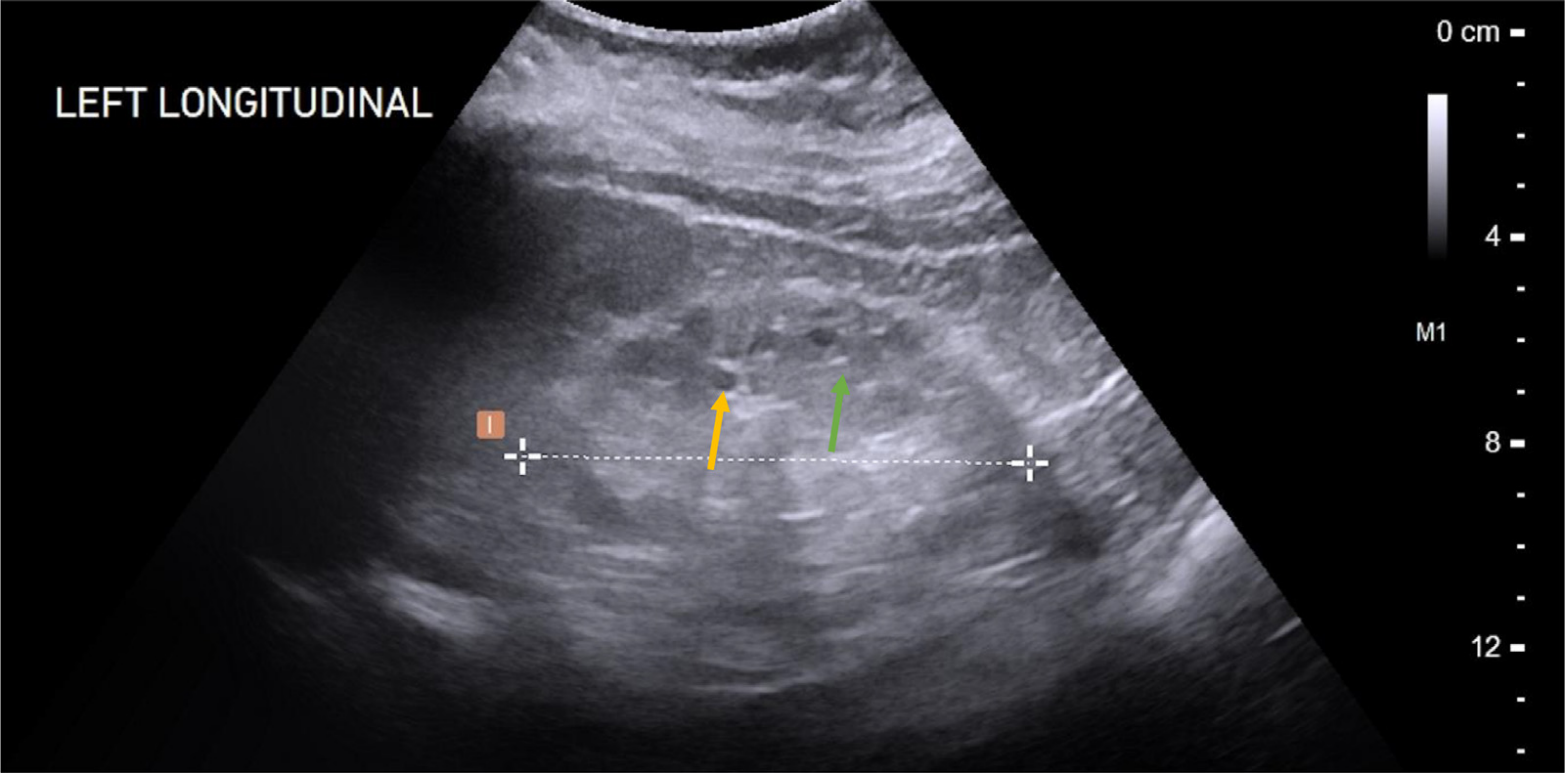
Point of care ultrasound performed in nephrology office of the left kidney. Multiple anechoic, well-circumscribed cystic structures and scattered echogenic foci are identified.
